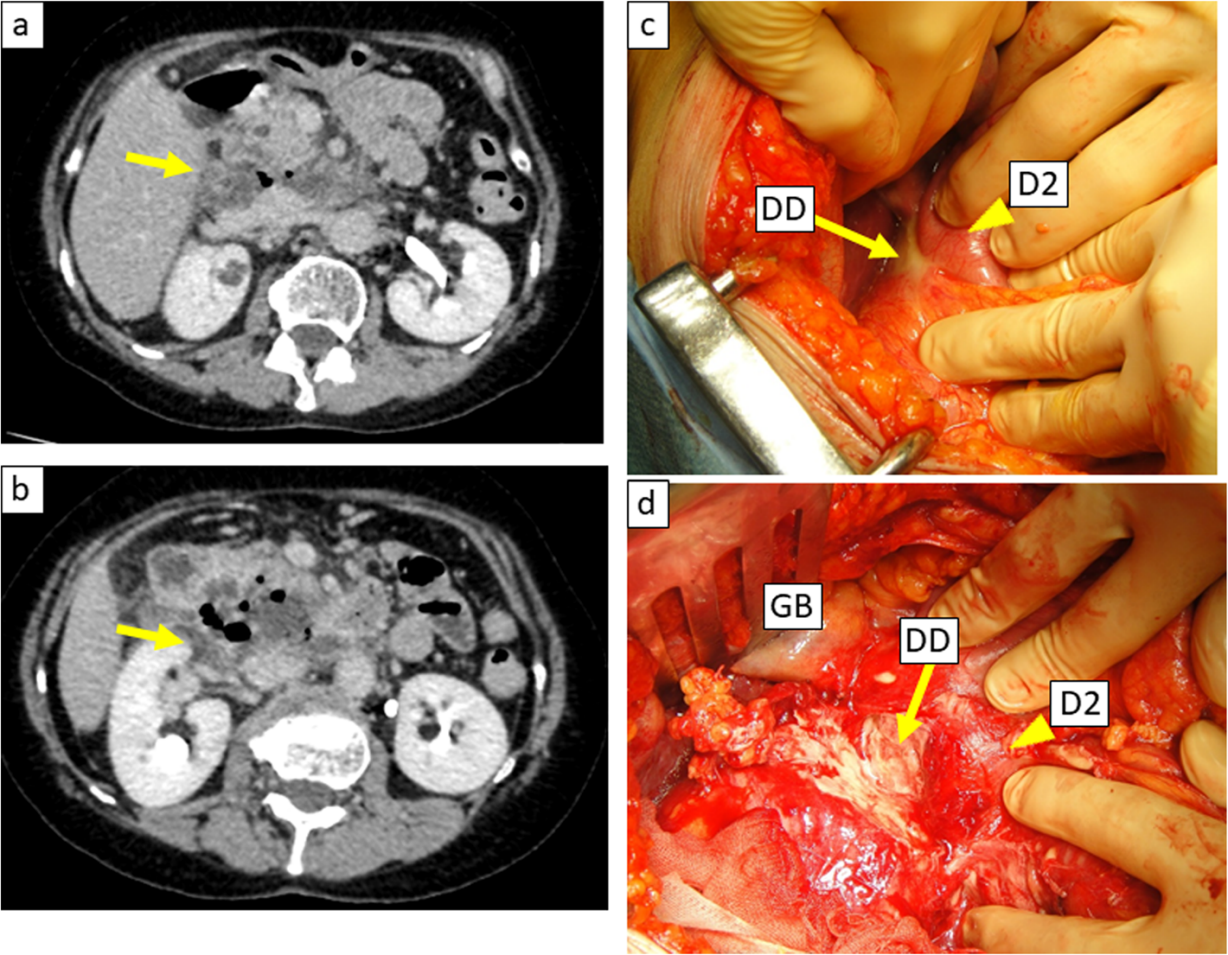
a, b Computed tomography images showing abscess with free air surrounding the second portion of the duodenum (yellow arrow). c, d Surgical findings of the perforation site. The perforated duodenal diverticulum (DD, yellow arrow) was identified on the dorsal side of the second portion of the duodenum (D2, yellow arrowhead; GB, gallbladder)
